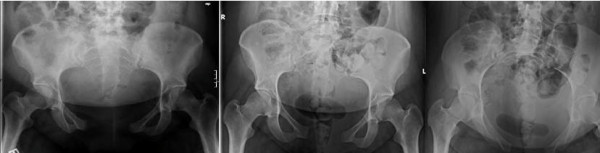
X-rays showing the symphysis pubis with 8.8 cm diastasis initially and 6.8 cm diastasis left with bilateral sacroiliac joint widening after one week of bed rest.
